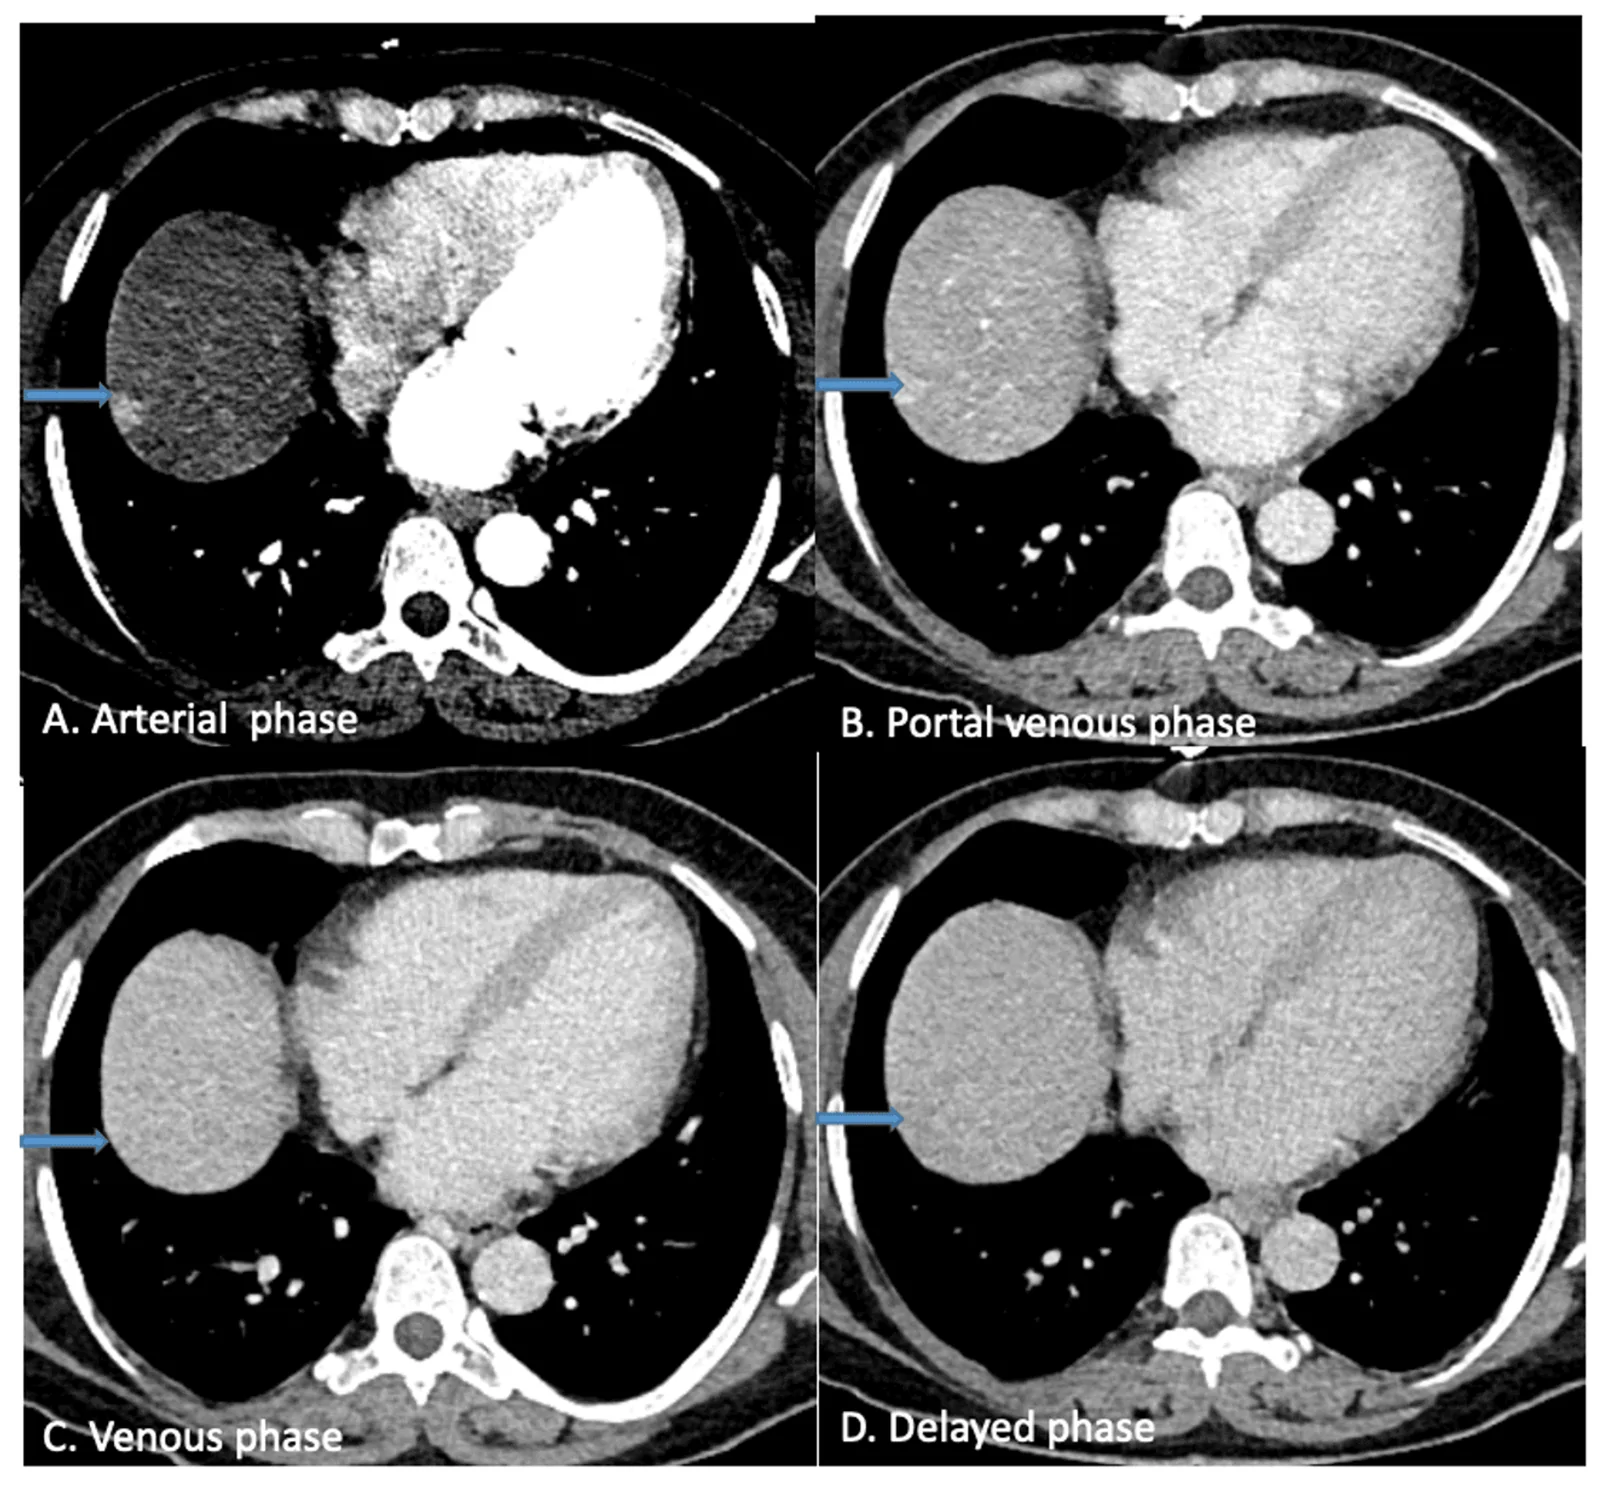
LR-3 observation on multiphasic contrast-enhanced CT. Axial multiphasic contrast-enhanced CT images obtained during the arterial (A), portal venous (B), venous (C), and delayed (D) phases demonstrate a well-defined hepatic observation measuring <10 mm (blue arrow) with nonrim arterial phase hyperenhancement. The observation demonstrates persistent enhancement on the portal venous phase and isoenhancement on the subsequent venous and delayed phases, without additional major or ancillary imaging features. In a patient with chronic liver disease, these imaging findings are consistent with an LR-3 observation according to the Liver Imaging Reporting and Data System (LI-RADS).
